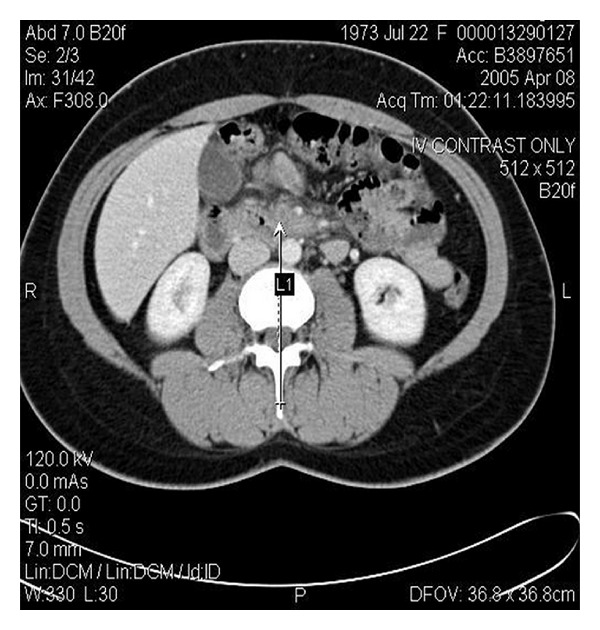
Contrasted abdominal computed tomography demonstrating partial thrombosis of the superior mesenteric vein as noted by the arrow.
